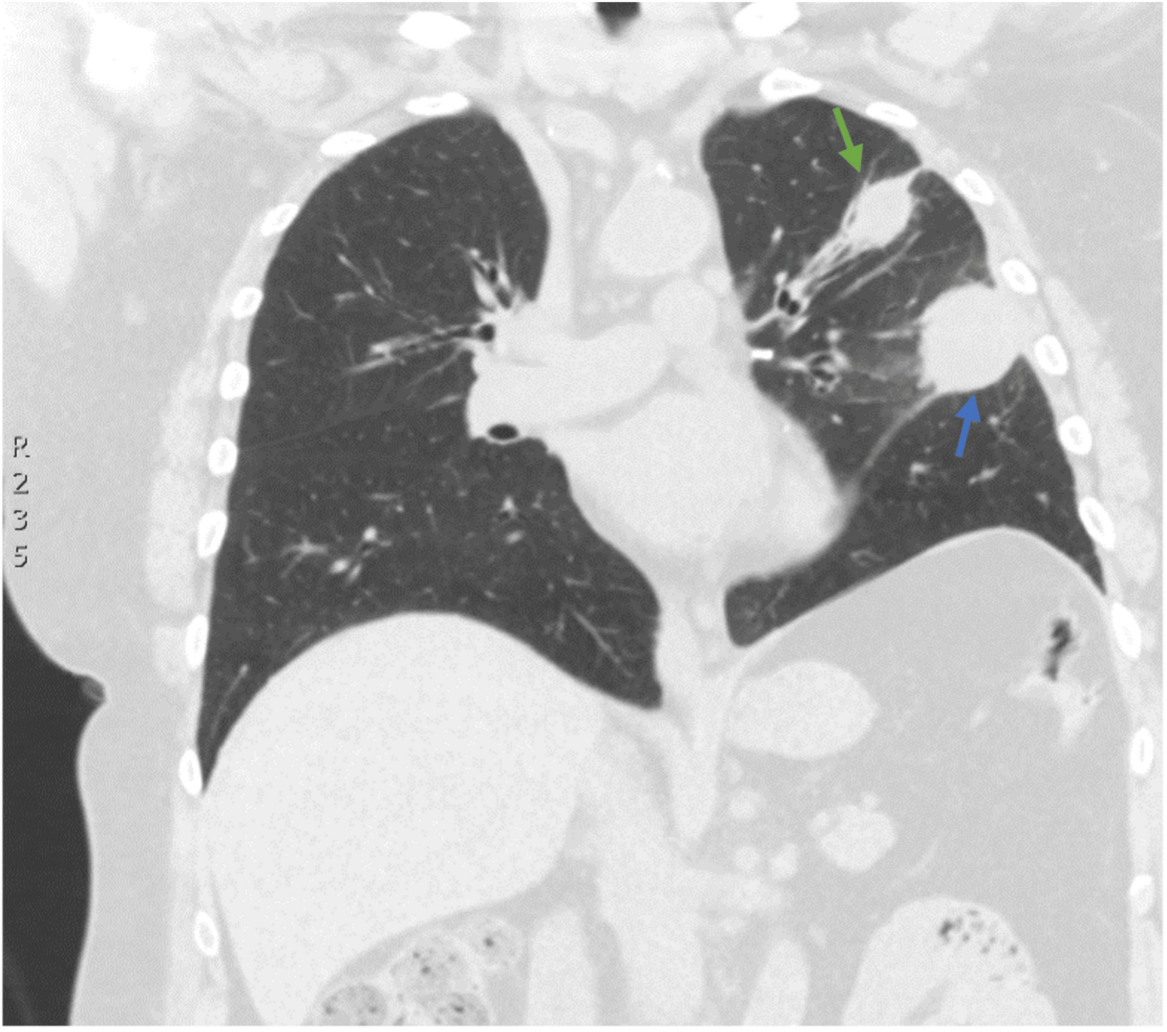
CT chest without contrast obtained during the early stages of the initial evaluation Coronal view demonstrating a mildly spiculated left upper lobe nodule, measuring 2.3x2x2 cm (green arrow), and a 4x3.5x3.5 cm mass invading the major fissure (blue arrow). Left-sided pleural thickening was also demonstrated. Slice thickness: 3 mm. Edge-enhanced reconstruction algorithm.
